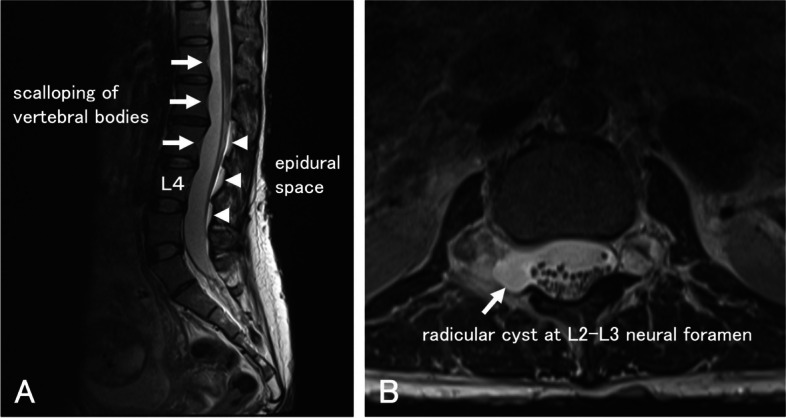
a Sagittal MRI showed widening of the dural sac and posterior scalloping of the vertebral bodies from L1 to L3 (arrows). Posterior segment of the epidural space was observed (arrow heads). b Axial MRI at L2-3 showed a radicular cyst expanding the right neural foramen (arrow)
